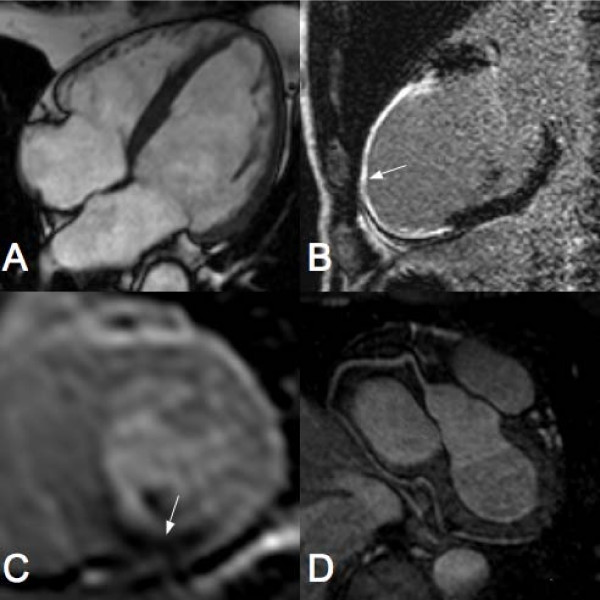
Sample cardiac MR images. A) Standard four chamber SSFP view of the heart LV showing both atria and ventricles. B) Vertical long axis late gadolinium enhancement view of the LV showing infarction of the anteroapical wall (arrow). C) Short axis view of the LV showing a perfusion defect in the inferior wall (arrow). D) Typical MR angiogram of a right coronary artery.
